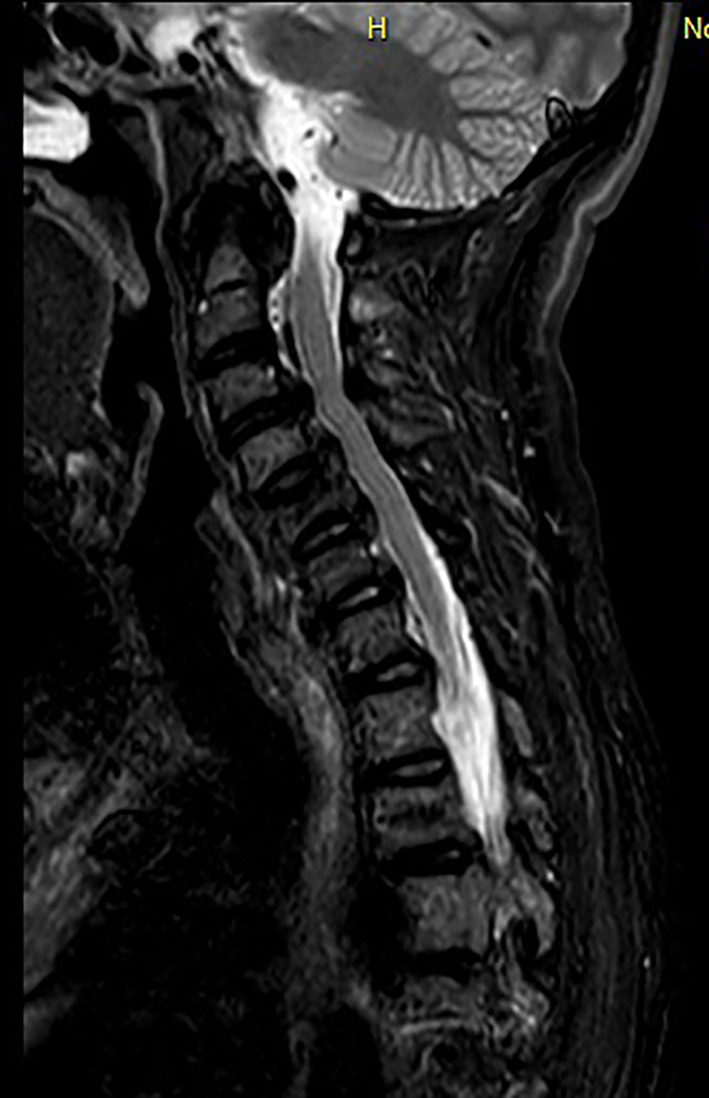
MRI of the cervical spine (March 2017). Sagittal sections, T2‐weighted sequences: extensive ossifications of the posterior longitudinal ligament and the annular ligament with a “crowned dens” appearance.
